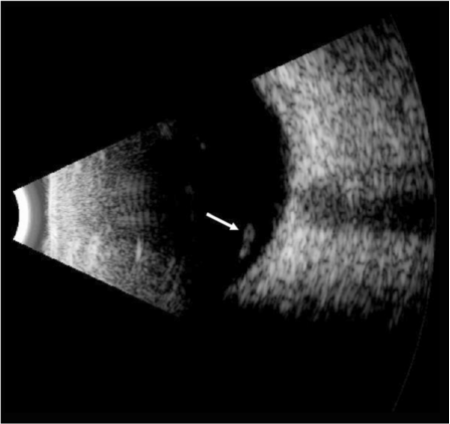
Ocular ultrasonography demonstrating the parasite (*white arrow*) within the vitreous. Note that the parasite is not in contact with the retina
